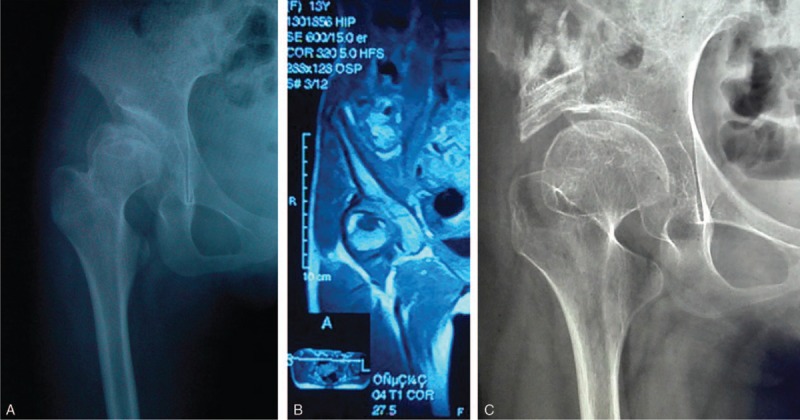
Radiological findings of a 13-year-old girl with a lesion of the right hip, treated with periosteal transplantation combined with surgical reduction of the femoral head and the augmentation of acetabulum. (A) Preoperative anteroposterior hip radiograph showing subluxation in right hip chondral defect in the anteromedial femoral head. (B) Preoperative MRI image was used to confirm location and size of the lesion. (C) Postoperative anteroposterior radiograph depicting well-repaired cartilage defects, good congruency between the femoral head and the shell and well preserved joint space.
